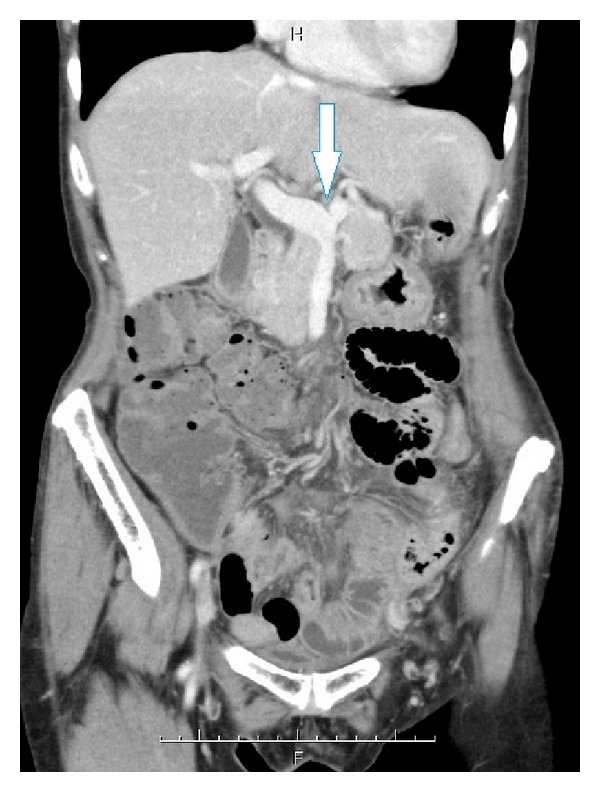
Contrast enhanced computed tomographic scan of the abdomen (coronal view) six days after starting anticoagulation therapy. The superior mesenteric vein is patent (arrow) and the thrombus has resolved.
